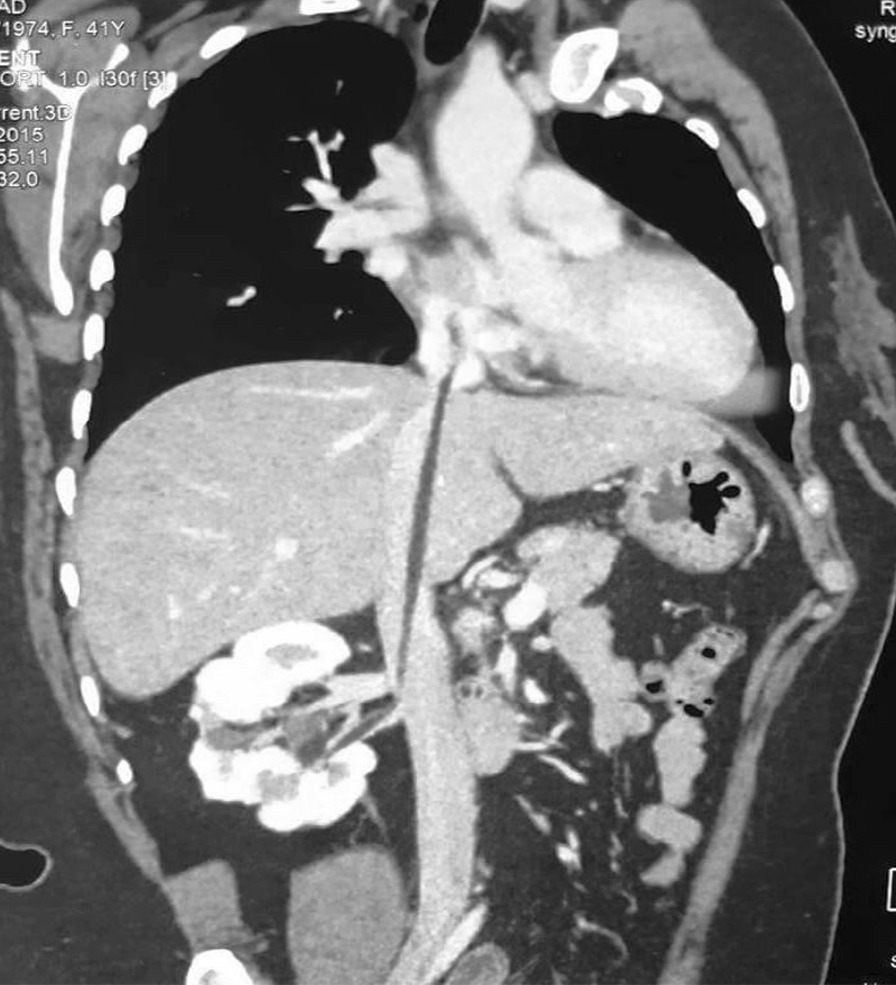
Computed tomography scan showing renal angiomyolippma with inferior vena cava thrombus. The thrombus extends up to the right atrium
